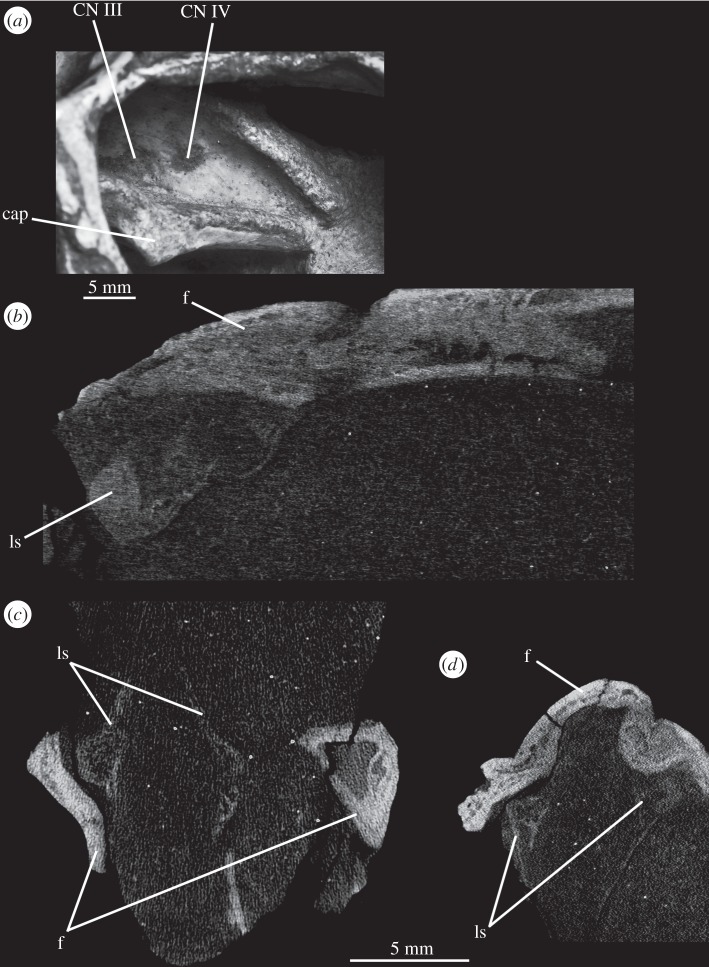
Laterosphenoid of SAM-PK-5867 (*a*) in left dorsolateral view, and CT slices on laterosphenoids of UMZC T.692 in (*b*) right lateral, (*c*) dorsal and (*d*) posterior view. For abbreviations, see table 1.
